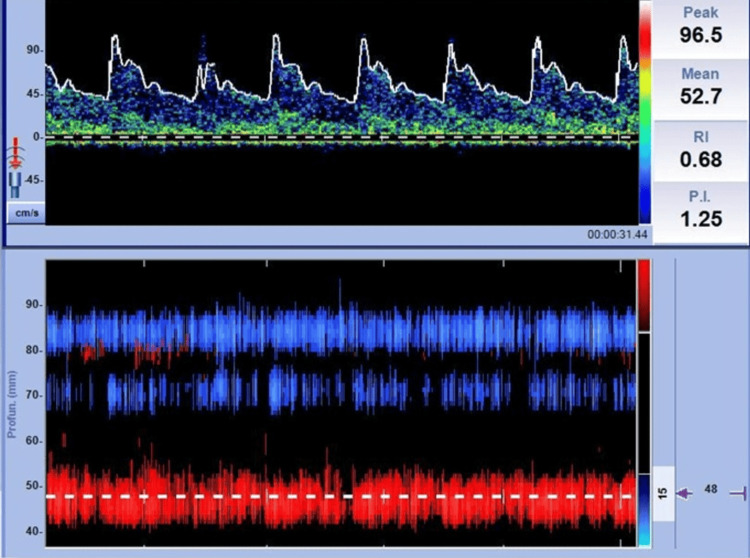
TCD of the MCA showing a PI of 1.25 and an RI of 0.68. TCD: Transcranial Doppler; MCA: Middle Cerebral Artery; PI: Pulsatility index; RI: Resistance index, Source: original work
